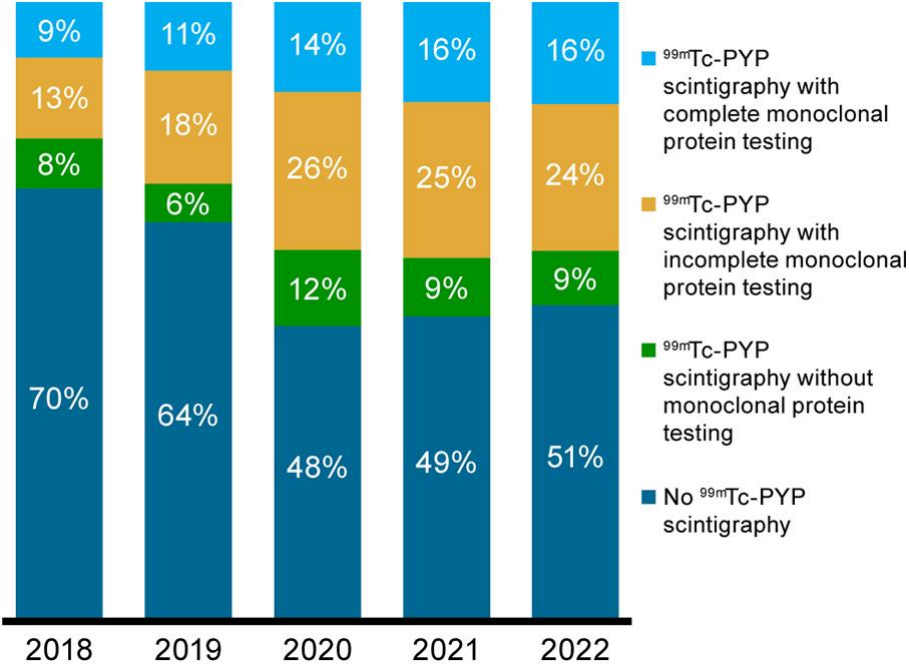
Proportions of patients without ^99m^Tc-PYP scintigraphy and with ^99m^Tc-PYP scintigraphy who had complete, incomplete, and no monoclonal protein testing for ATTRwt-CM in the 2-year period before ATTRwt-CM diagnosis by diagnosis year. ^99m^Tc-PYP, technetium-99m pyrophosphate scintigraphy; ATTRwt-CM, wild-type transthyretin amyloid cardiomyopathy.
